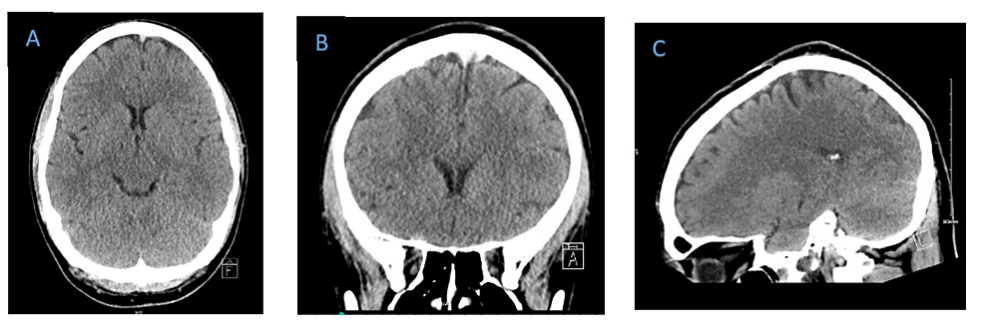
CT of the head done on admission A: axial view; B: coronal view; C: sagittal view
